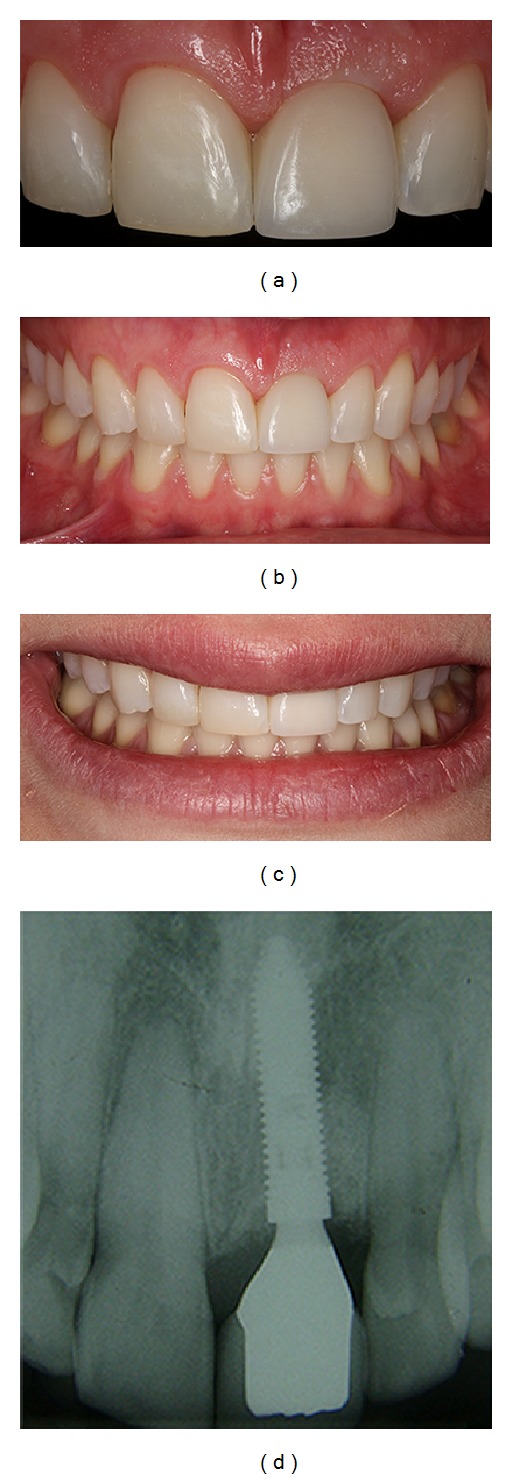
(a, b, c, d) Postoperative clinical and radiographic views, immediately after the conclusion of the prosthetically restored maxillary right central incisor.
